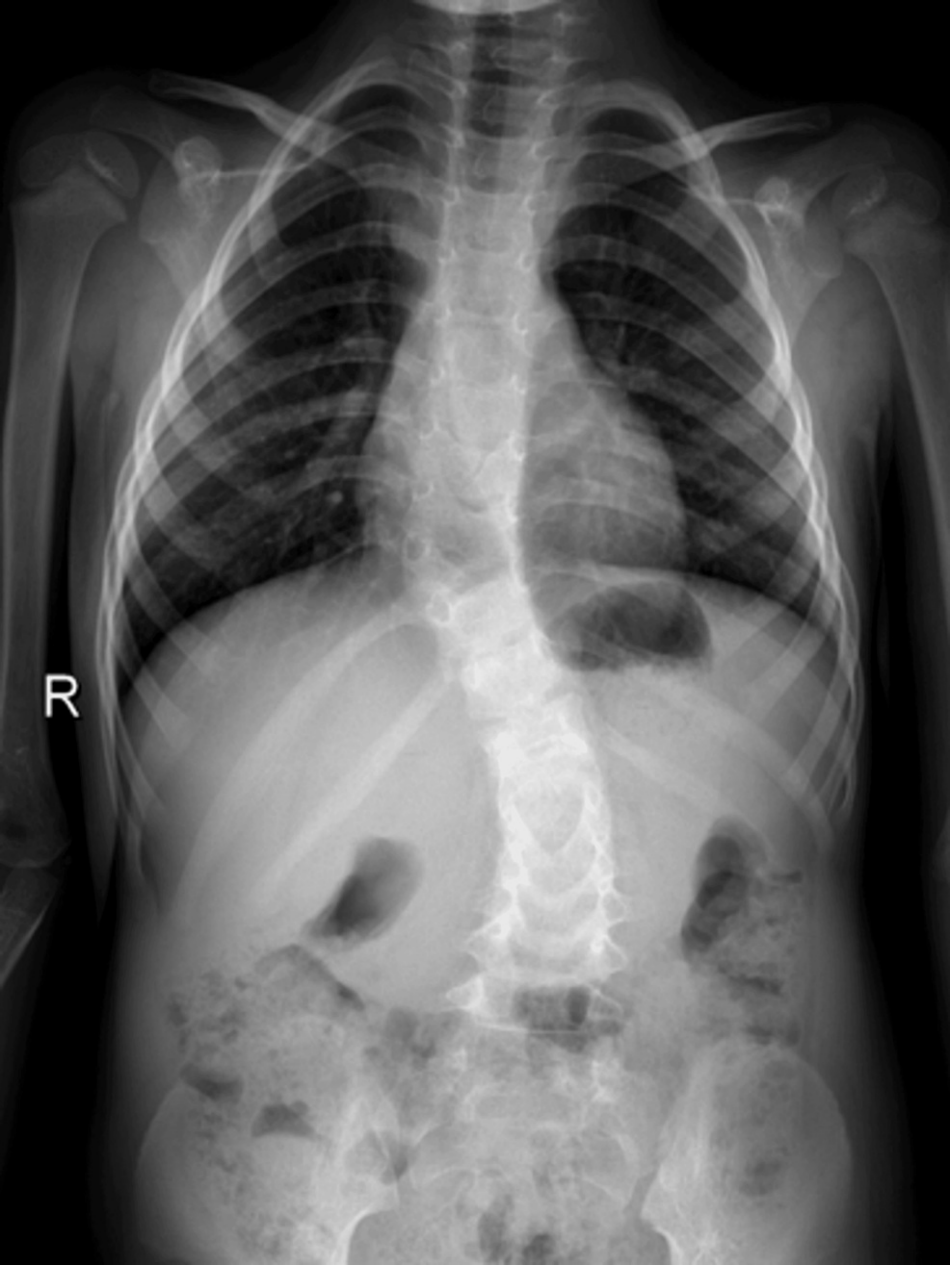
A pre-operative anteroposterior radiograph of the thoracolumbar spine depicts the 70-degree angle of congenital kyphosis and the two hemivertebrae of T10 and T12.
